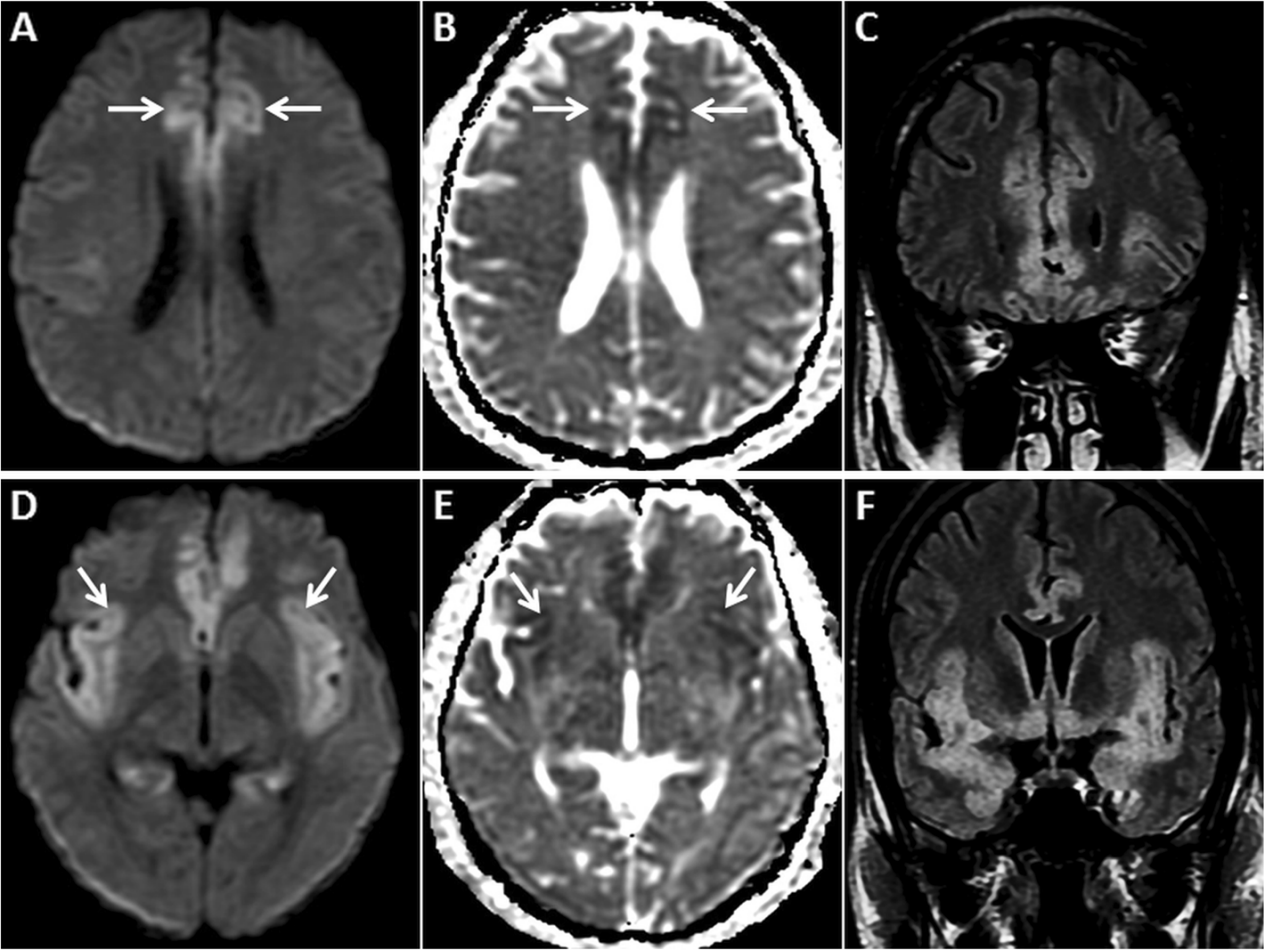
MRI of the brain in a 26-year-old female patient brought unconscious following valproate overdose. Symmetric GRD is seen in the cingulate gyri (arrows) on axial DWI (a) and ADC image (b). GRD is also seen in the insular cortices (arrows) on axial DWI (d) and ADC image (e). Coronal FLAIR images (c, f) reveal hyperintense cortical swelling in these regions, along with similar signal changes in bilateral mesial temporal lobes. Laboratory tests confirmed the diagnosis of hyperammonaemia
